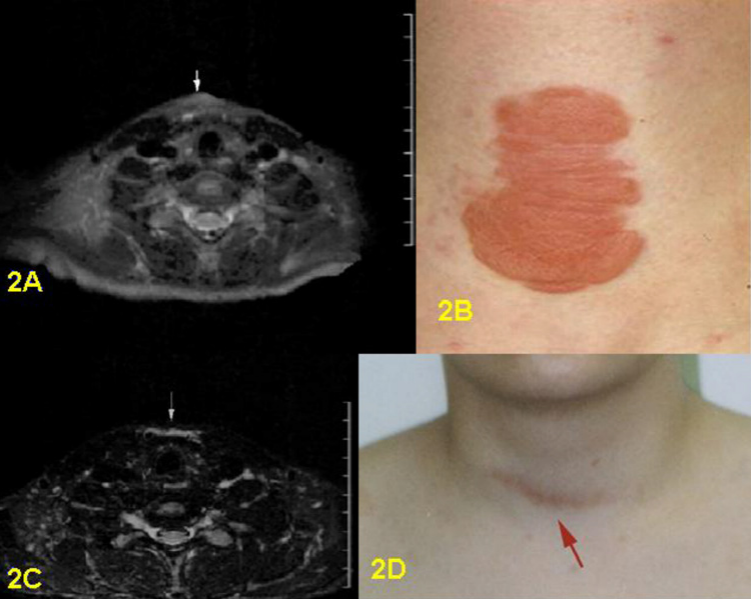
2A: Pre-surgical T2-weighted STIR-sequence, axial section: superficial tumor covering the laryngeal prominence. 2B: Pre-surgical clinical frontal view of PNF of ventral neck. 2C: Post-surgical T2-weighted STIR-sequence, axial section: scar tissue praelaryngeal visible. 2D: Post-surgical clinical frontal view of laryngeal scar.
